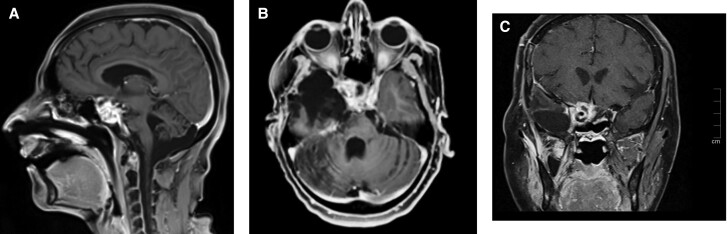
Contrast-enhanced T1 magnetic resonance imaging dynamic pituitary scan (A, sagittal; B, axial; C, coronal sections) reveals postoperative changes with residual enhancing tumor in the right lateral sella cavity with extension into the right cavernous sinus and parasellar region encasing the cavernous and inferiorly extends through the foramen ovale below the skull base up to approximately 1.5 cm. Anteriorly, it extends up to the right orbital apex and posteriorly extends along the right dorsal surface of clivus.
